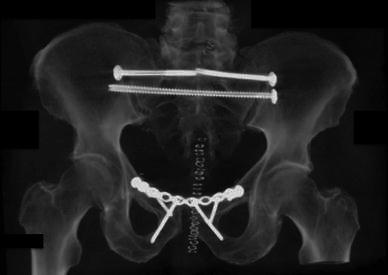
Three-dimensional reconstruction image from the postoperative CT scan of a patient with a pelvic ring injury who had pubic symphysis fixation with a 10-hole 3.5-mm pelvic reconstruction plate placed through a vertical midline incision
